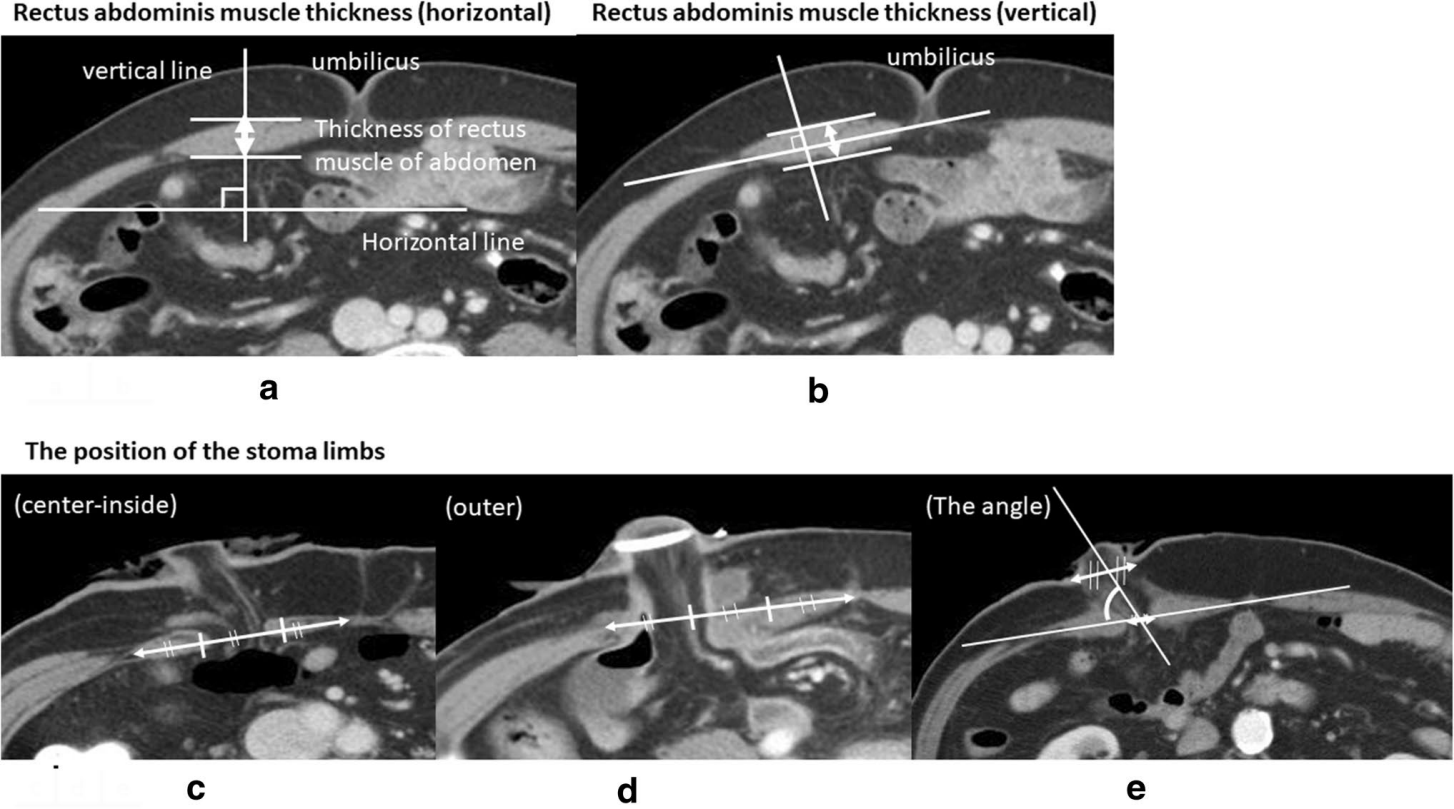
a A straight line is drawn perpendicular to the horizontal axis at the thickest part of the right rectus abdominis muscle. b A straight line is drawn perpendicular to the straight line connecting both ends of the rectus abdominis muscle. c The stoma limbs are classified as penetrating the center-inside. d The stoma limbs are classified as penetrating the outside. e The angle formed by the straight line connecting both ends of the rectus abdominis muscle and the long axis of the stoma limbs is measured on a CT scan image and on the slice in which the stoma limbs penetrate the rectus abdominis
